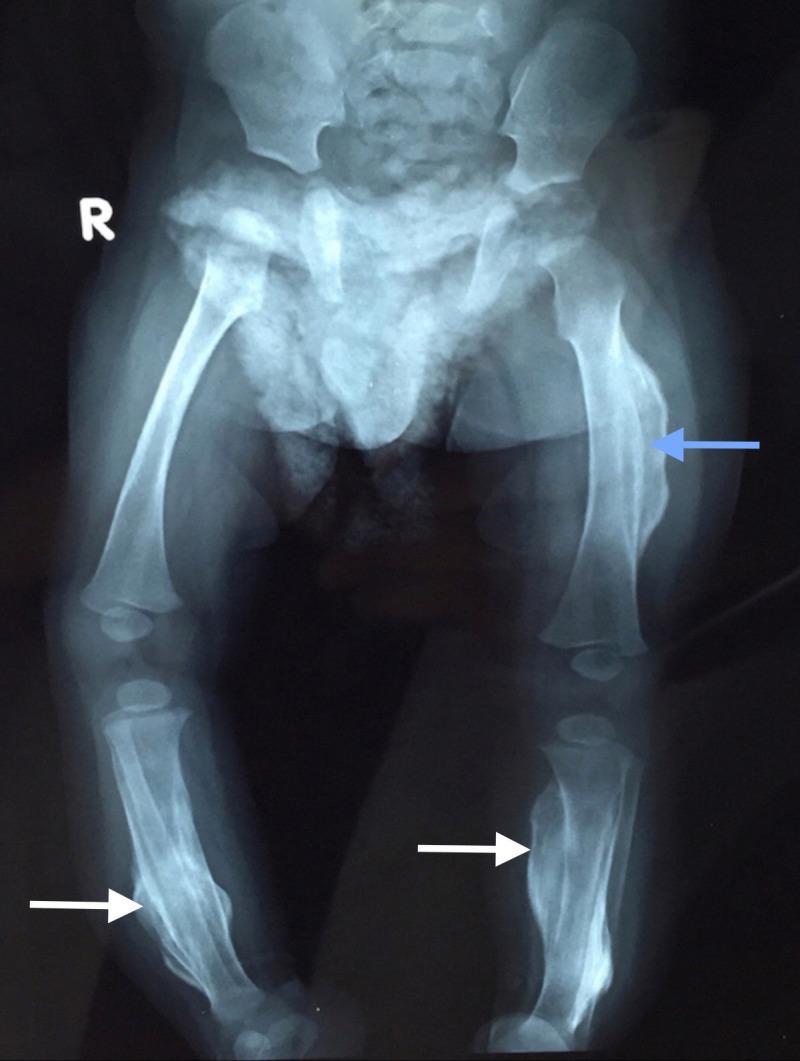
Anteroposterior (AP) view of bilateral lower limbs Radiograph of a 5-month-old male showing complete encasement of left femur by thick lamellated periosteal new bone (blue arrow) confined to diaphysis with epiphyseal and metaphyseal sparing. Similar lamellated two fusiform new bone is also involving diaphysis of bilateral tibia (white arrows).
